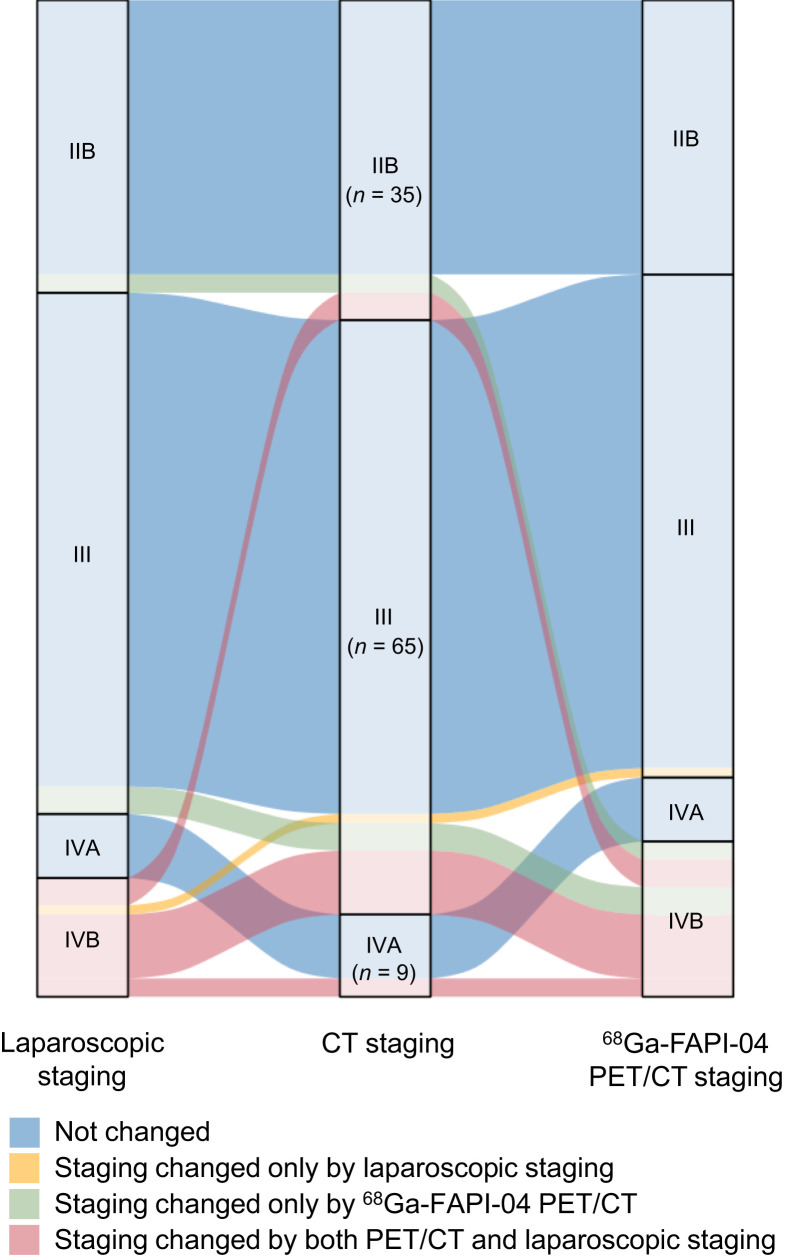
Visualization of inner layers of staging showed metastatic staging of patients changed after FAPI PET/CT examination and laparoscopic staging. Nodes on far left represent metastatic staging changed by laparoscopic staging. Nodes in second layer represent initial staging based on CT. Final layer represents metastatic staging changed by FAPI PET/CT. Staging changed only by FAPI PET/CT in 17 patients(15.6%), of whom 5 (4.6%) changed from IIB to IVB, 10 (9.2%) changed from III to IVB, and 2 (1.8%) changed from IVA to IVB. Of these 17 cases, 12 (70.6%) were identified as peritoneal metastases by laparoscopic staging. Only positive peritoneal cytology (CY1) was found in 3 of 17 patients.
